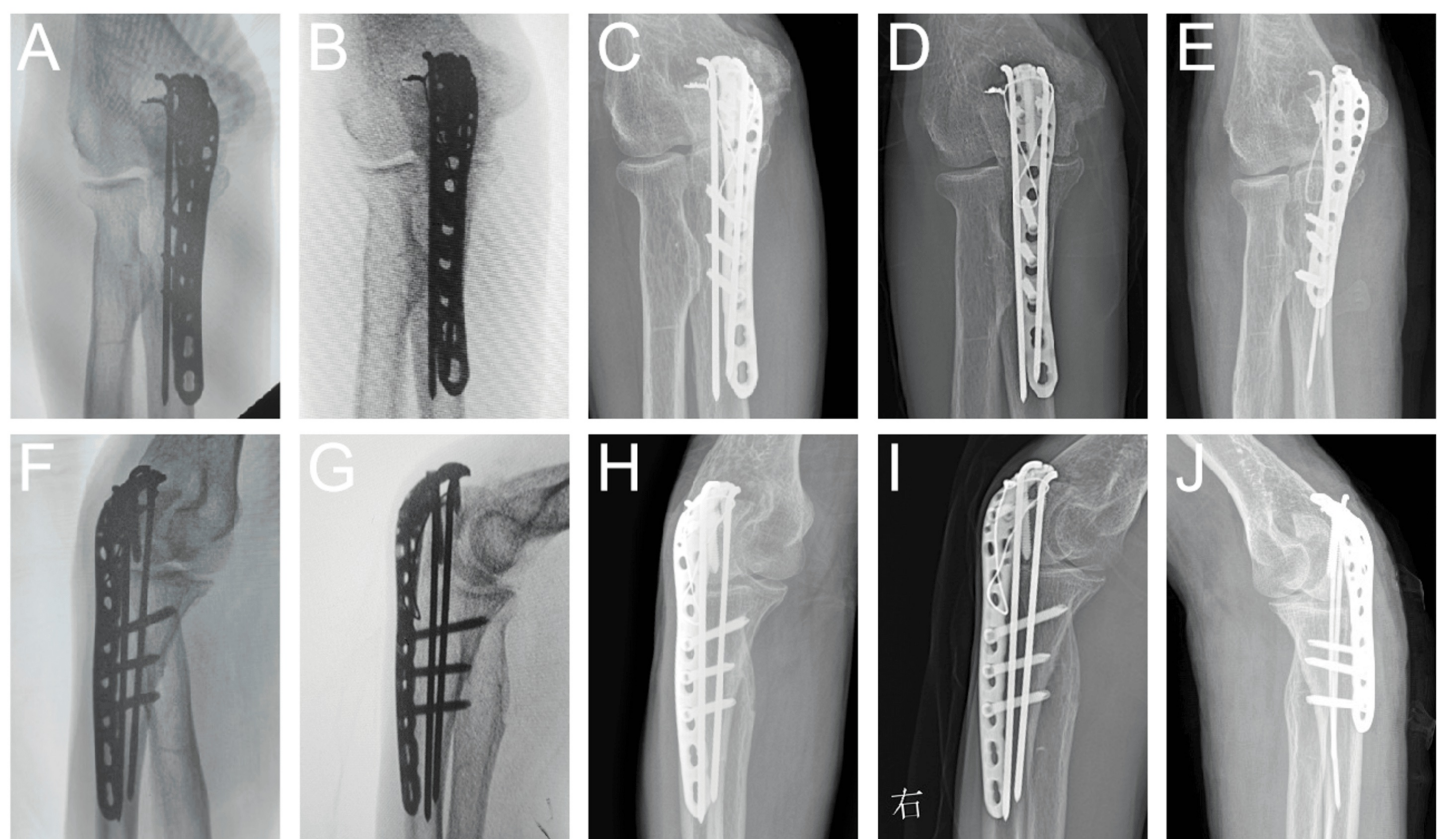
Series of follow-up radiographs. (A, F) One month postoperatively, anteroposterior and lateral projections of the right elbow; (B, G)Three months postoperatively, anteroposterior and lateral projections of the right elbow; (C, H)Five months postoperatively, anteroposterior and lateral projections of the right elbow; (D, I)Eight months postoperatively, anteroposterior and lateral projections of the right elbow; (E, J) Nearly three months postoperatively, anteroposterior and lateral projections of the left elbow.
